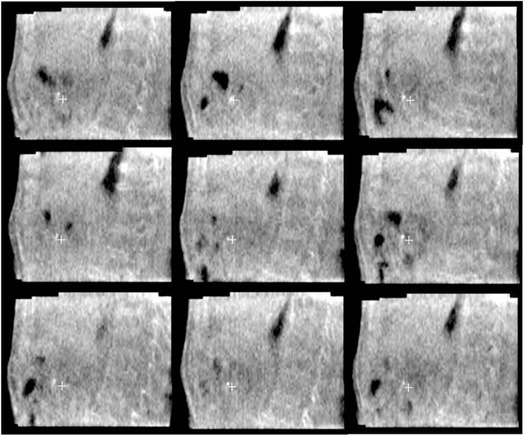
Sagittal views of MV‐CBCT for nine different treatment sessions in patient #8. The position of the fiducial in the first MV‐CBCT is shown (symbol ‘+’).
